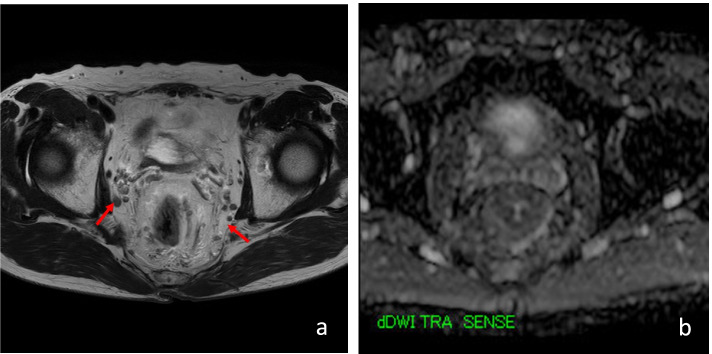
T2 and DWI MRI examinations showed pelvic lymph node swelling (red arrowhead)
